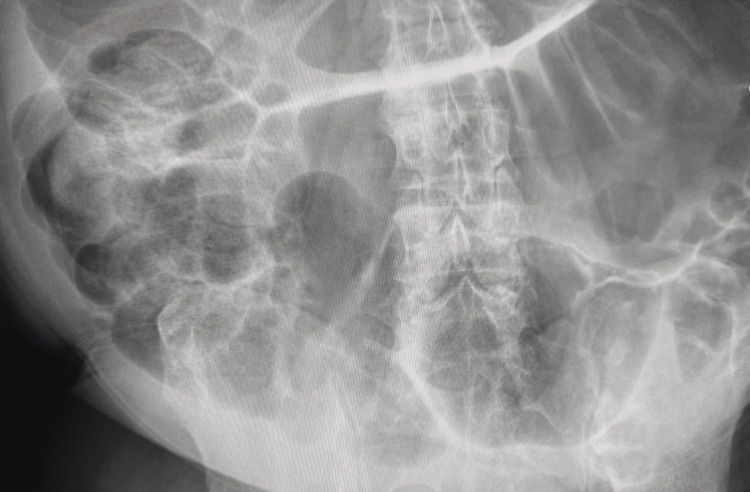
Additional kidney, ureter, bladder (KUB) radiograph in rehabilitation, taken from a different angle, also demonstrated significant colonic dilation without an obstructive transition point. The repeated finding across views reinforces the diagnosis of pseudo-obstruction rather than a focal blockage.
